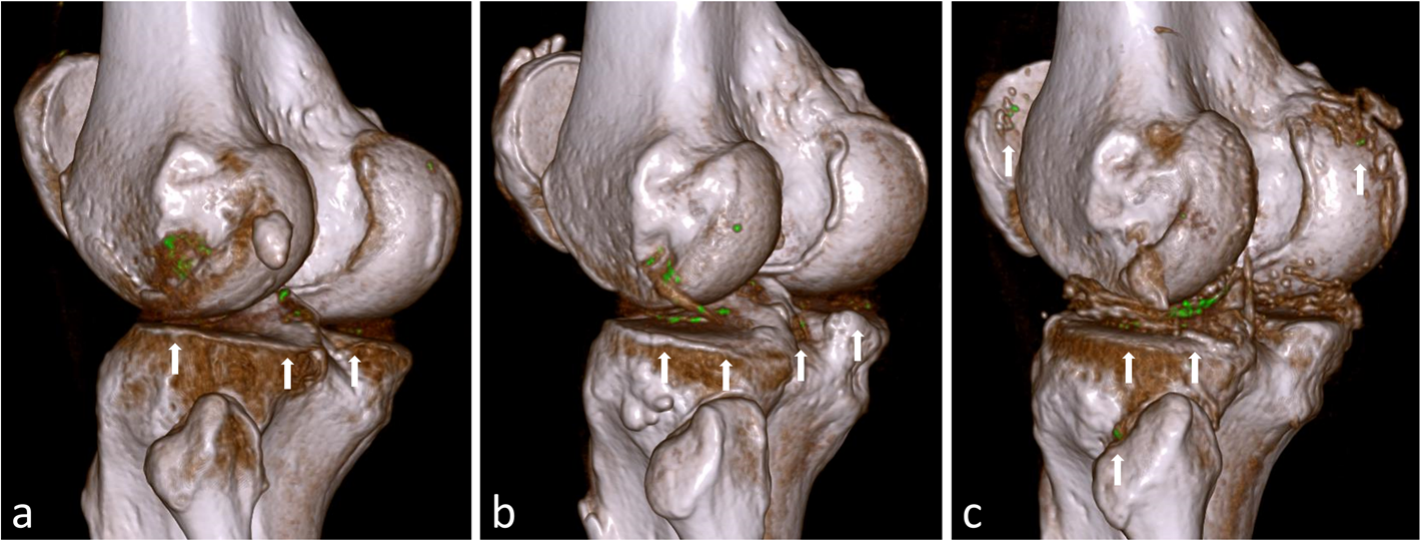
DECT images showing examples of colour-coded DECT lesions in knee joints. a–c Dual-energy CT (DECT) scans of the left knee joints in three different patients showing colour-coded DECT lesions with similar appearances and locations. Analysis of the DECT ratios revealed that a is dominated by *definite MSU depositions*, while b and c are dominated by likely calcium-containing depositions. a
